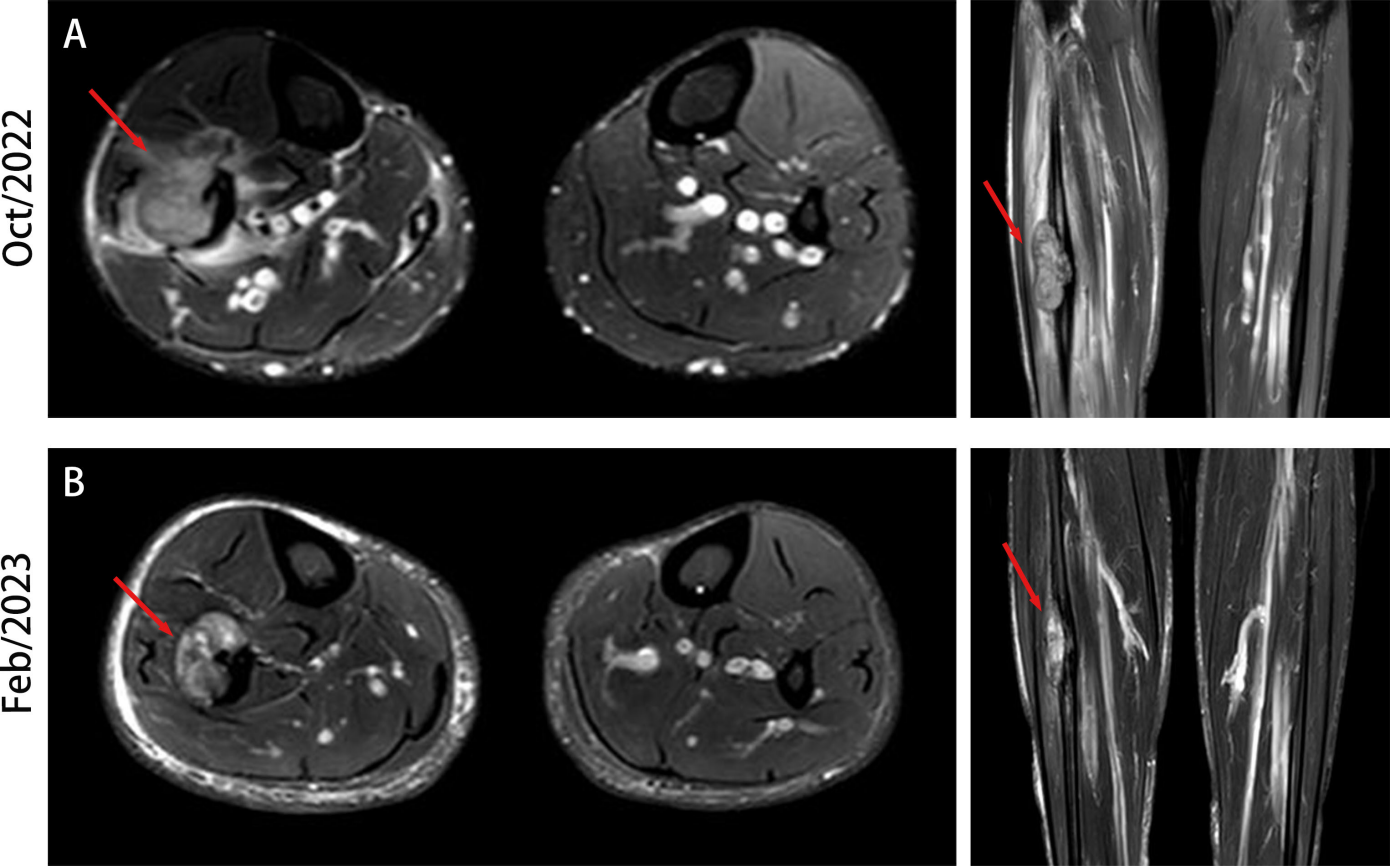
MRI of fibular lesion. (A) MRI highlights of fibular lesion at the time of initial diagnosis. (B) MRI presentation of fibular lesion after two cycles of treatment.
